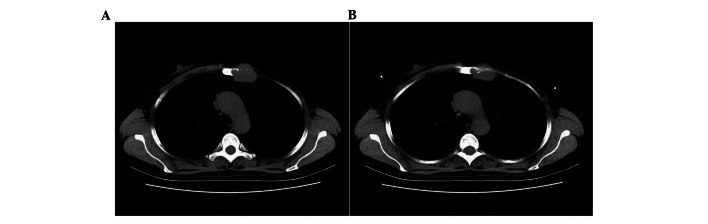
Chest computed tomography (CT) images. (A and B) reveal bone destruction of the left sternum and a 3.4×3.5-cm mass that intruded the left chest cavity.
